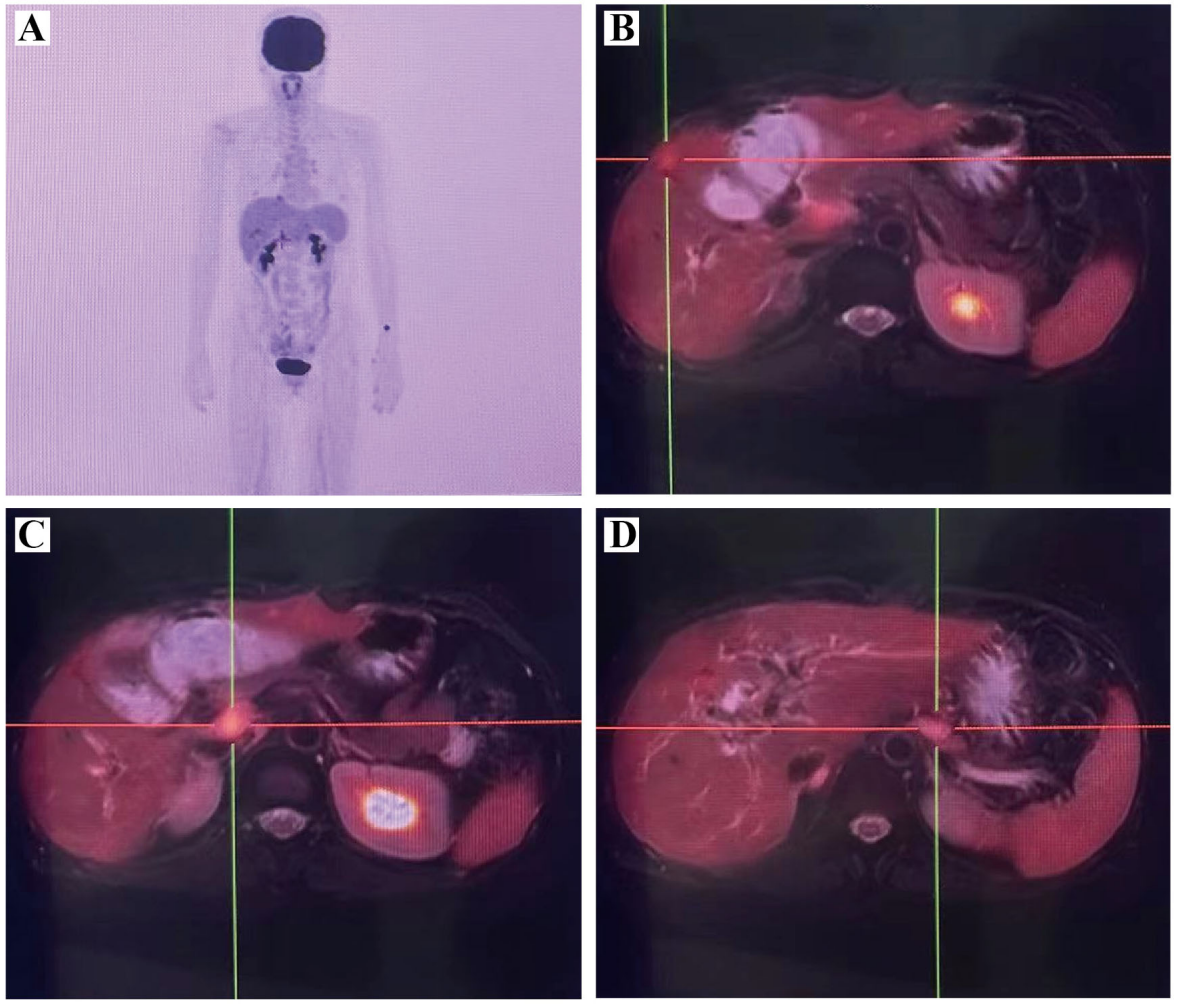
PET/MRI suggested recurrence and metastasis of gallbladder carcinosarcoma. (A) PET MIP image; (B) Multiple metastases in liver; (C) Lymph node metastasis in the hepatoportal area; (D) Lymph node metastasis in the gastric omental area. PET/MRI, positron emission tomography/magnetic resonance imaging.
